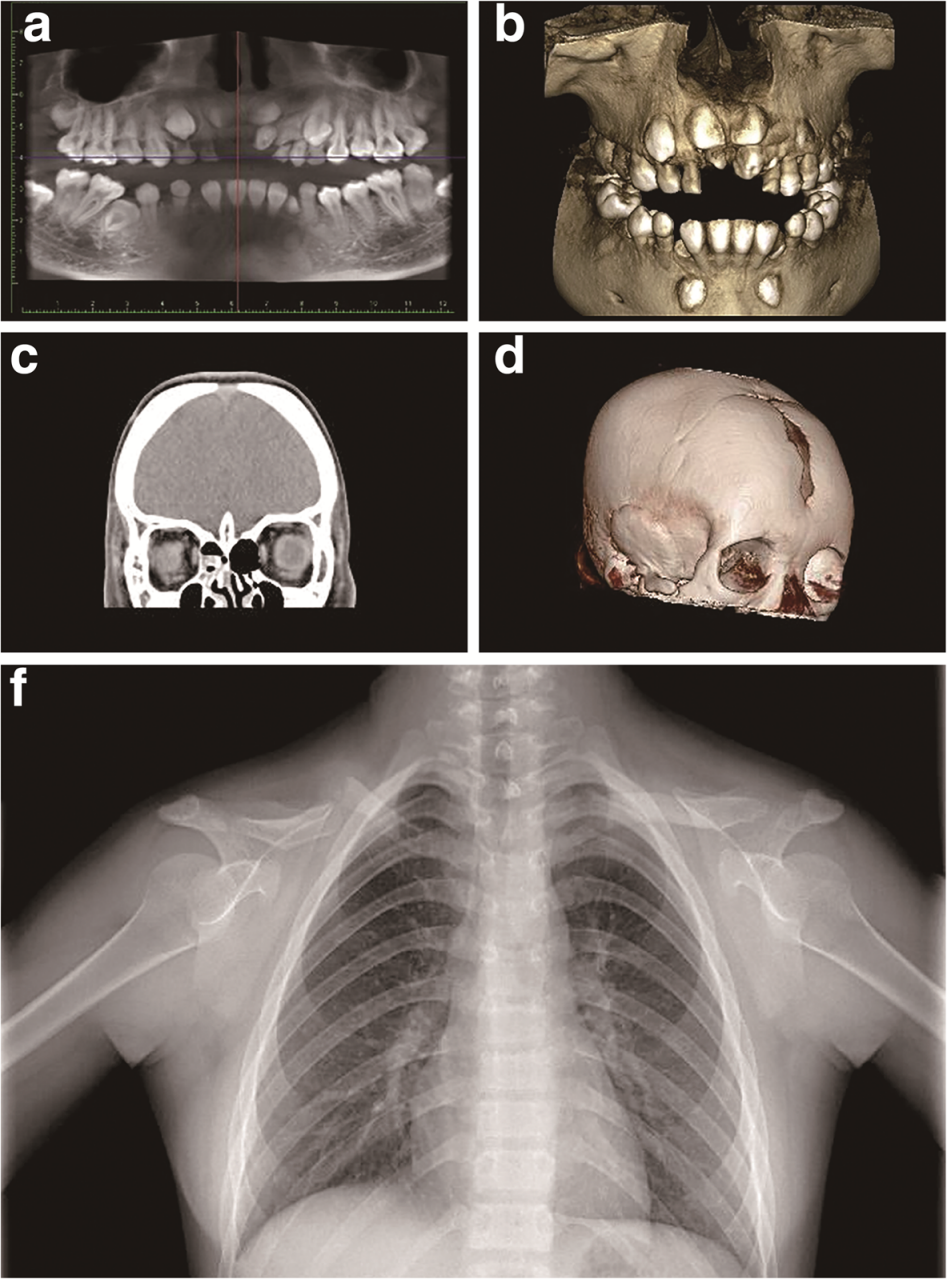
Radiological findings for the patient. a, b Cone-beam computed tomography results showing detailed dental abnormalities, including impacted supernumerary teeth, the retention of primary teeth, eruption failure of the permanent teeth, and impaired root development. c, d A skull CT scan showed the presence of open fontanelles. e Radiographs revealed hypoplastic or aplastic distal ends of clavicles and structural abnormalities occurring in the right shoulder peak joint
